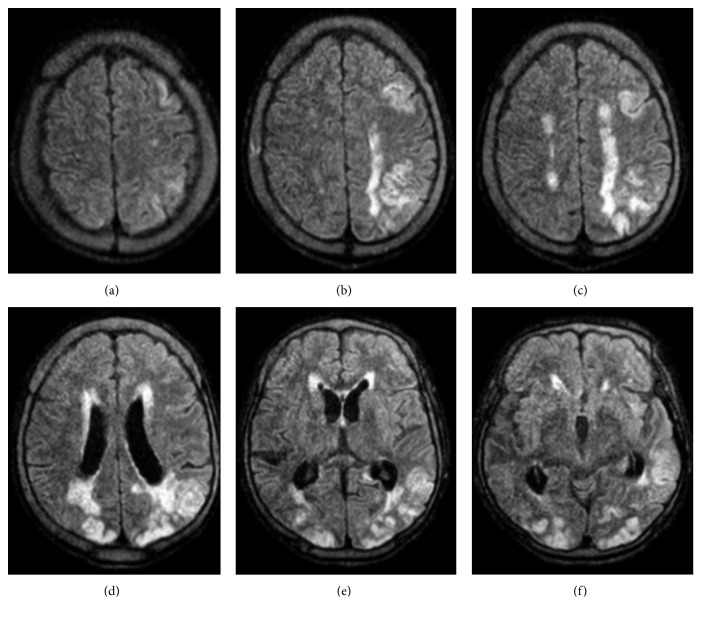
T2-weighted MRI without contrast images of the brain. The hyperintense areas reflect areas of ischemia. These were reviewed as recent infarcts in the ACA, MCA, and PCA territories. No intracranial hemorrhage can be identified. The radiologist also noted a mild mass effect with no midline shift.
